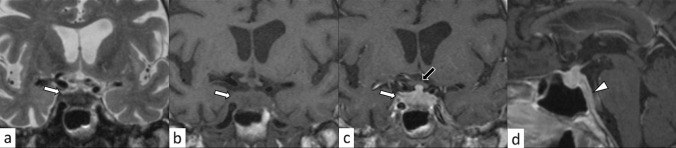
A 76-year-old woman with plasmacytic/IgG4-related hypophysitis. An enlarged strongly enhanced pituitary gland and stalk are observed (a–c, white arrows). The lesion shows heterogeneous hypointensity on T2-weighted coronal image a and hypo- to isointensity on T1-weighted coronal image b with involvement of the right cavernous sinus and compression of the optic chiasm (c, black arrow). Inflammatory extension along the dorsal surface of the clivus is observed (d, arrowhead)
